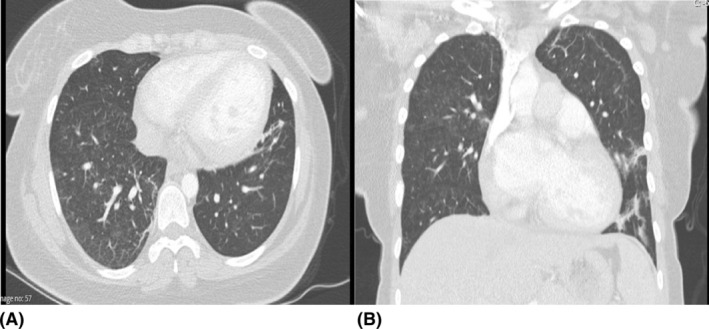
Repeat CT scan 3 weeks post commencement of therapy shows near complete resolution of diffuse consolidations.
